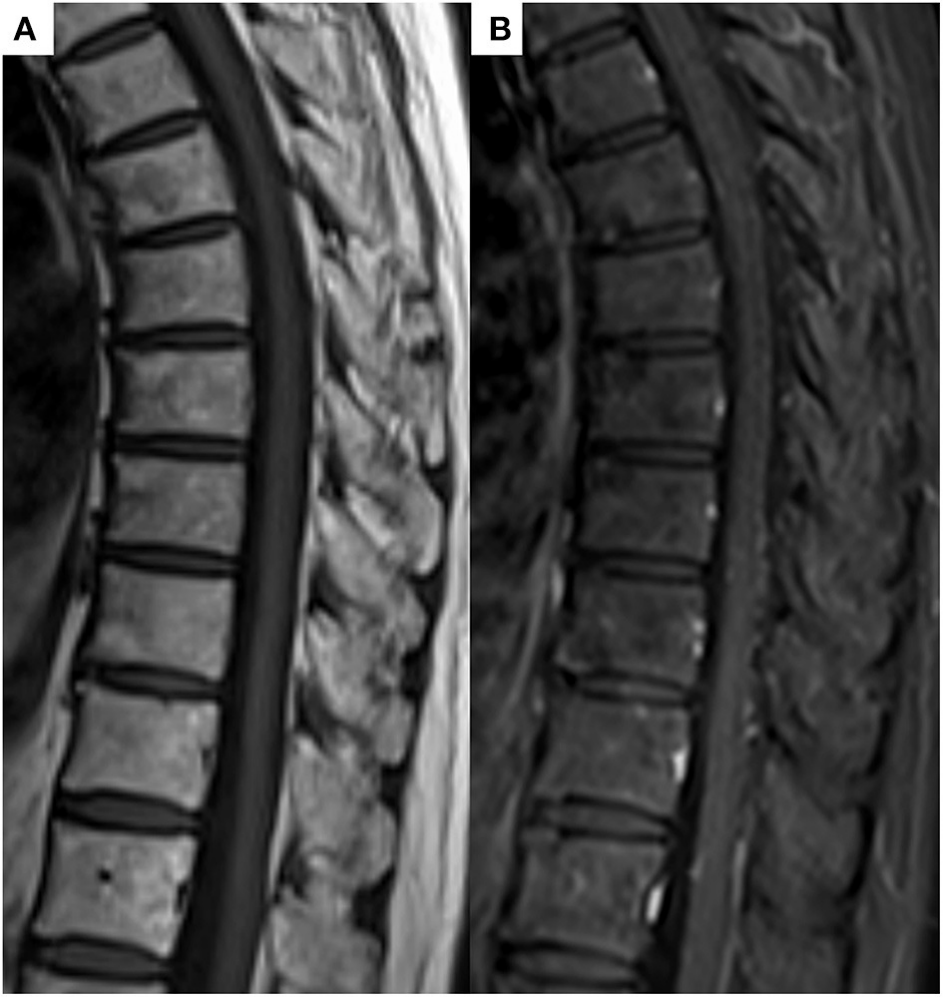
(A) Follow-up T1-weighted image and (B) contrast T1-weighted image after 5 months showed complete resolution of all previously noted bony lesions.
